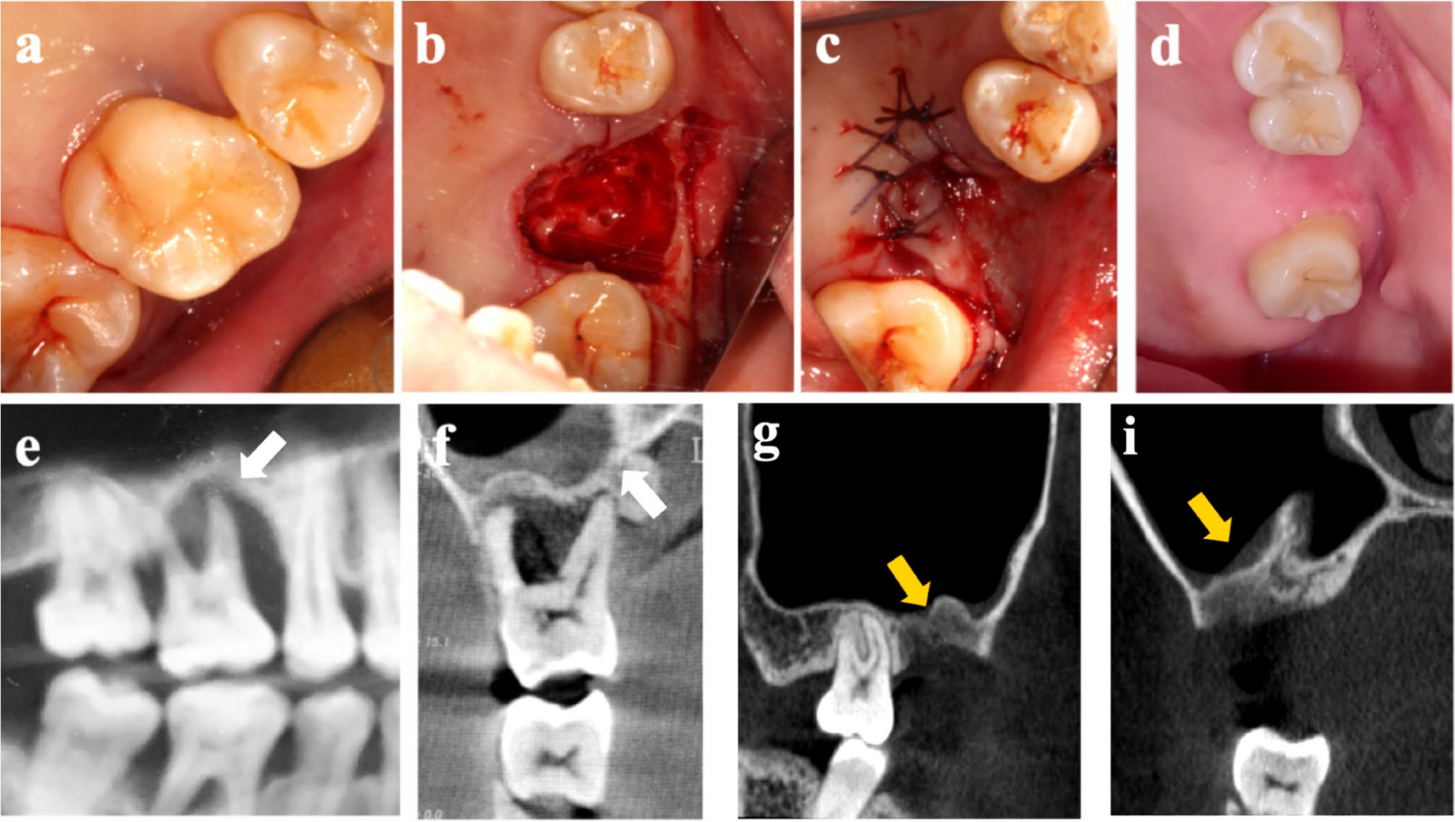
Clinical (a, b, c, d) and Imaging examination (e, f, g, i) of an 28-year-old female with breast cancer treated with zoledronic acid. Clinically, the maxillary first molar exhibited severe periodontitis, and no evidence of exposed bone was observed during clinical evaluation (a), the tooth was extracted using the Mucoperiosteal flap combined with perforation technique (b, c), and 3 month later, the extraction socket demonstrated complete mucosal healing without clinical signs of infection or inflammation (d). In sagittal (e) and coronal (f) CBCT views, angular bone resorption of the proximal mesial up to the root apex and inflammation of the mucosa at the floor of the maxillary sinus are pointed out by white arrows. CBCT images obtained 3 months postoperatively demonstrated new bone formation at the extraction socket in sagittal (g) and substantial regression of inflammation region of the mucosa at the floor of the maxillary sinus in coronal (i) CBCT views depicted by yellow arrows
